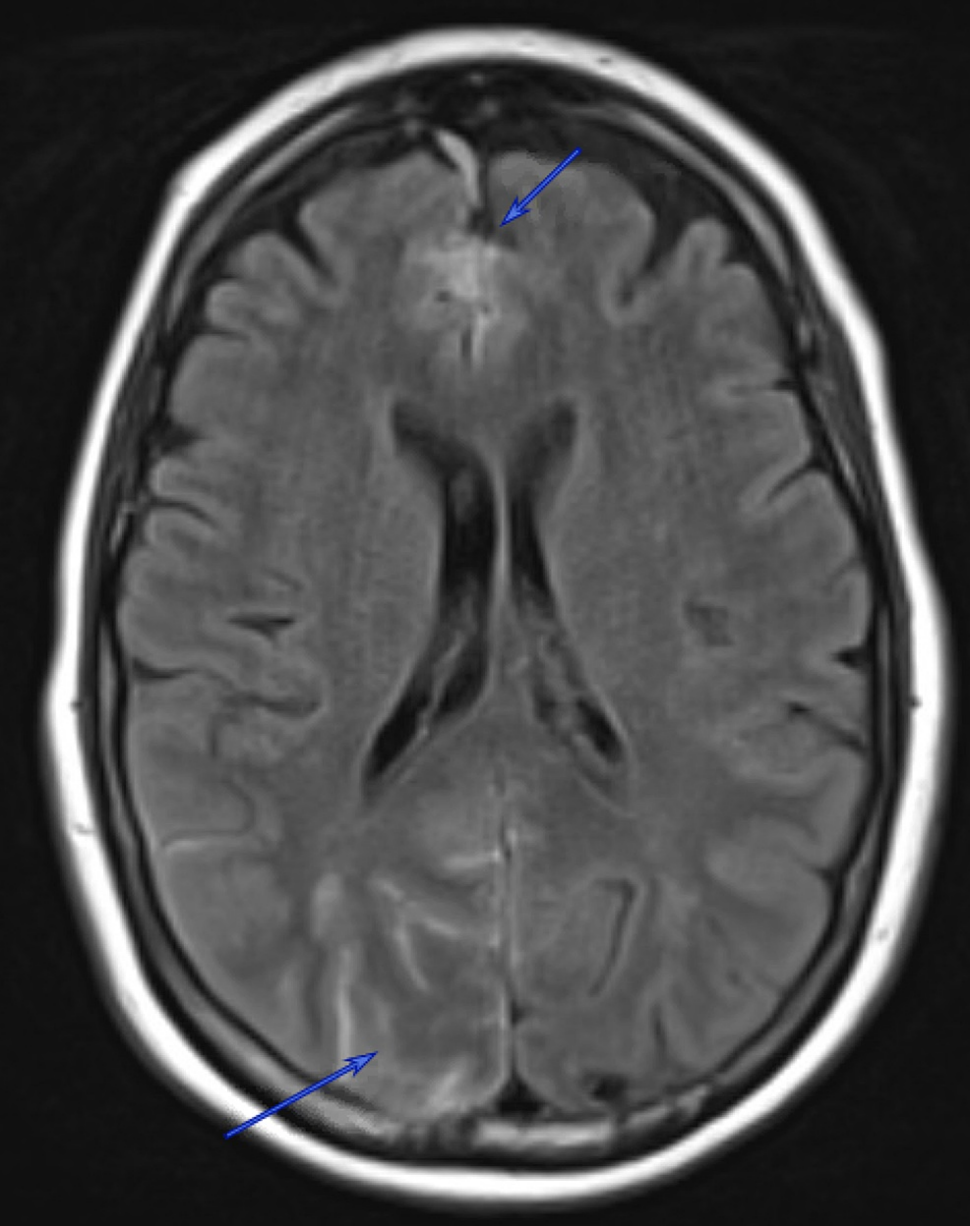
Multifocal Convexity Subarachnoid Hemorrhage Fluid-attenuated inversion recovery (FLAIR) sequence demonstrates two distinctive areas of subarachnoid hemorrhage (blue arrows).
